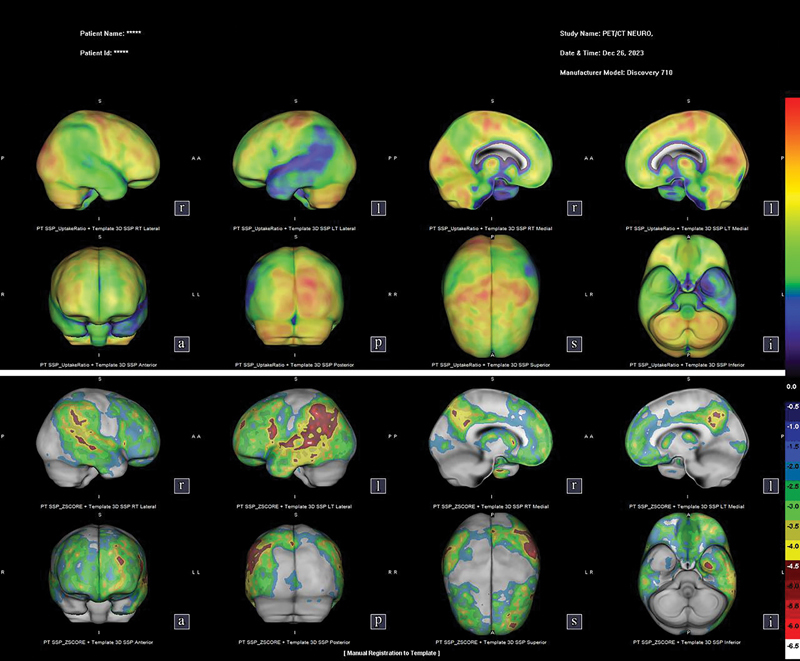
Case 1 - FDG PET scan showing glycolytic hypometabolism in the bilateral temporoparietal cortex, more pronounced on the left, suggestive of Alzheimer's disease.
